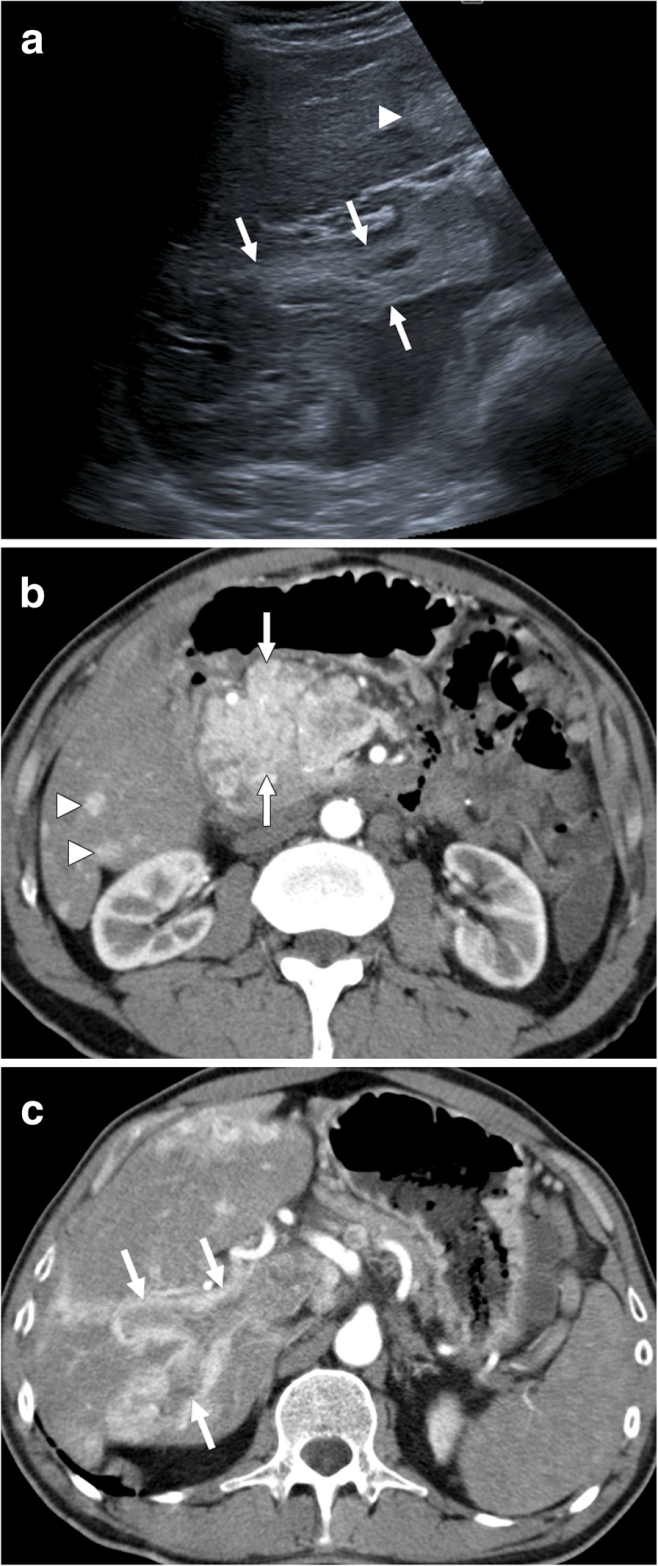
A 55-year-old male with no past medical history presented with epigastric pain and severe weight loss. a Gray-scale ultrasound image demonstrated a large mass in the epigastric area (not shown) with hyperechoic thrombus within the main portal vein (arrows). There was also a focal solid lesion within the left liver lobe (arrowhead) suggestive for metastatic disease. b Axial plane postcontrast arterial phase CT image shows avidly enhancing mass (arrows) located within the pancreatic head. Imaging findings were highly suggestive for pancreatic neuroendocrine tumor (NET). Also, note is made of small enhancing nodules within the liver parenchyma suggestive for metastases (arrowheads). c Axial plane postcontrast arterial phase CT image shows enlarged right portal vein with its lumen filled with contrast enhancing tumor thrombus (arrows). Percutaneous image-guided biopsy from the mass revealed pancreatic NET
